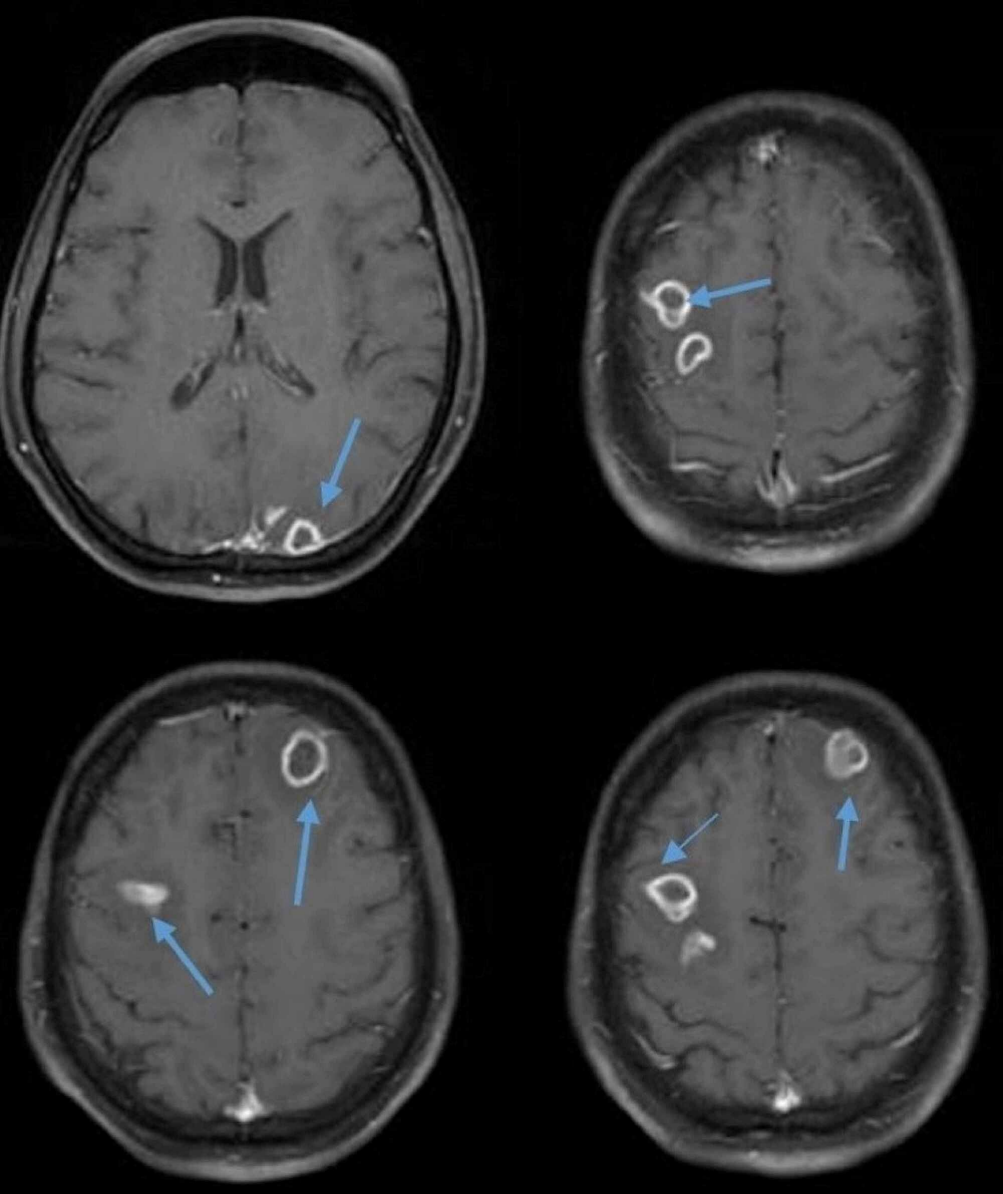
Axial images of brain MRI showing multiple ring-enhancing lesions of various sizes in the frontal, parietal, and temporal lobe (blue arrows). MRI: Magnetic resonance imaging
